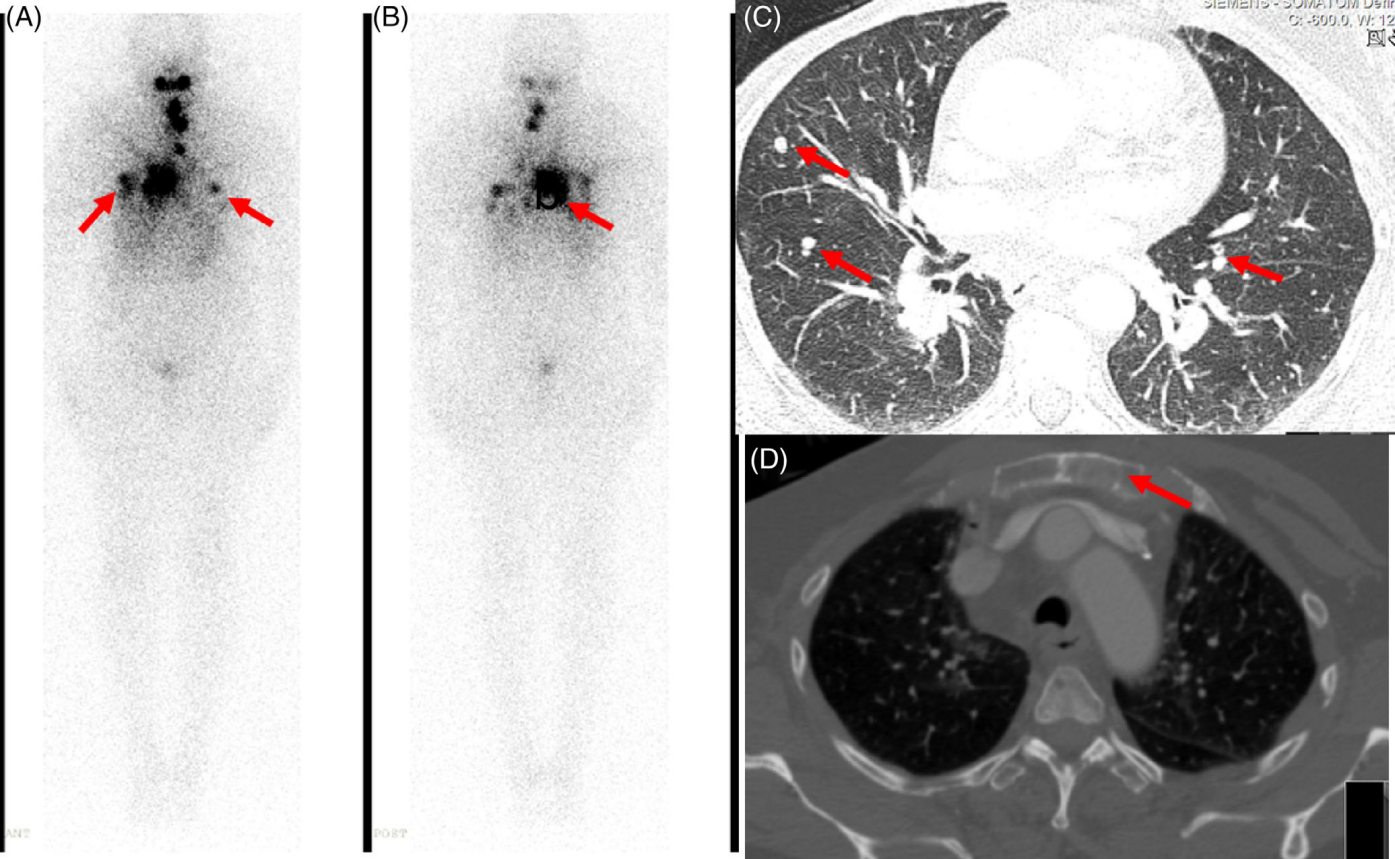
Iodine 131 nuclear study. Iodine 131 nuclear study (A) demonstrates the presence of multiple foci of abnormal tracer accumulation in the lungs (arrows) representing metastasis. (B) Increased tracer uptake in the sternum is also noted. (C) Pulmonary metastatic nodules were visualized on the CT chest (arrows). (D) A lytic sternum metastatic lesion (arrow) corresponds to the increased tracer uptake
